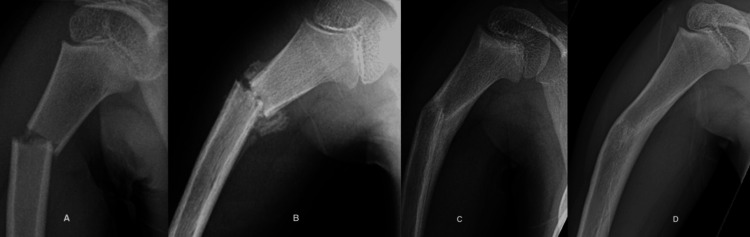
A proximal humeral fracture in a seven-year-old girl showing the phases of fracture healing. A. The initial radiograph shows angulation and displacement of the fracture. B. Three weeks post-injury with a cuff of soft callus.  Note more callus in the convexity. C. Three months after the fracture showing woven bone in the concavity and a smooth cortex on the convexity due to osteoclast activity.  D. One year after the fracture showing restoration of the medullary canal and the cortices.
